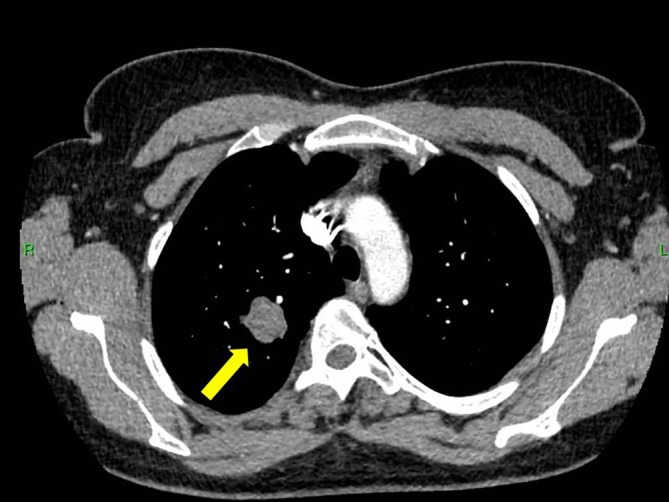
Chest CT angiography showing a malignant tumor in the right upper lobe of the lung (yellow arrow), further characterizing the thoracic lesion prior to surgical management.
